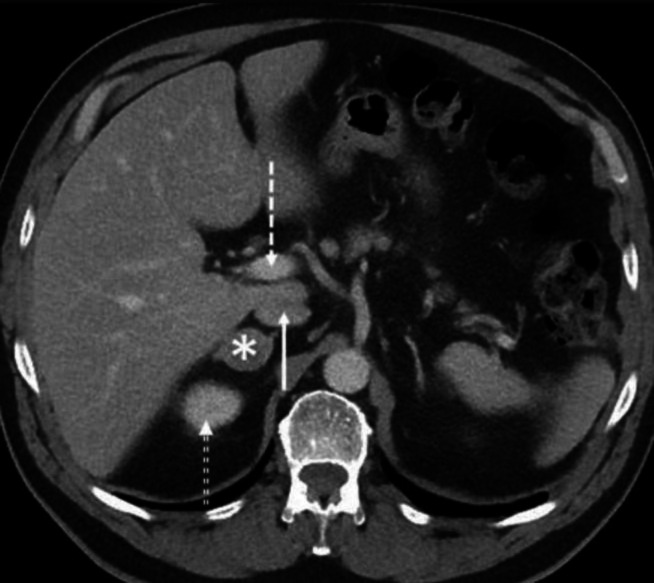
Contrast enhanced axial CT abdomen of a 70-year-old male who presented with clinical features suggestive of acute diverticulitis shows a well-defined, homogenous, low density lesion (asterisk) with mild enhancement in the right upper abdomen. The lesion is located posterior to the IVC (solid white arrow) and superior to the right kidney (double white arrow) suggesting it is originating from the right adrenal gland. As the density of lesion was measured to be 6HU, it most likely represents an adrenal adenoma. Adrenal adenoma is often found incidentally during abdominal imaging and has low density due to presence of intracytoplasmic lipid. On a non-contrast CT an adrenal lesion with attenuation of <10HU is considered highly specific for an adrenal adenoma. Note that CT attenuation of a lipid poor adenoma can be >10HU necessitating further workup (e.g., contrast enhanced CT or magnetic resonance imaging) for catherization of the lesion
